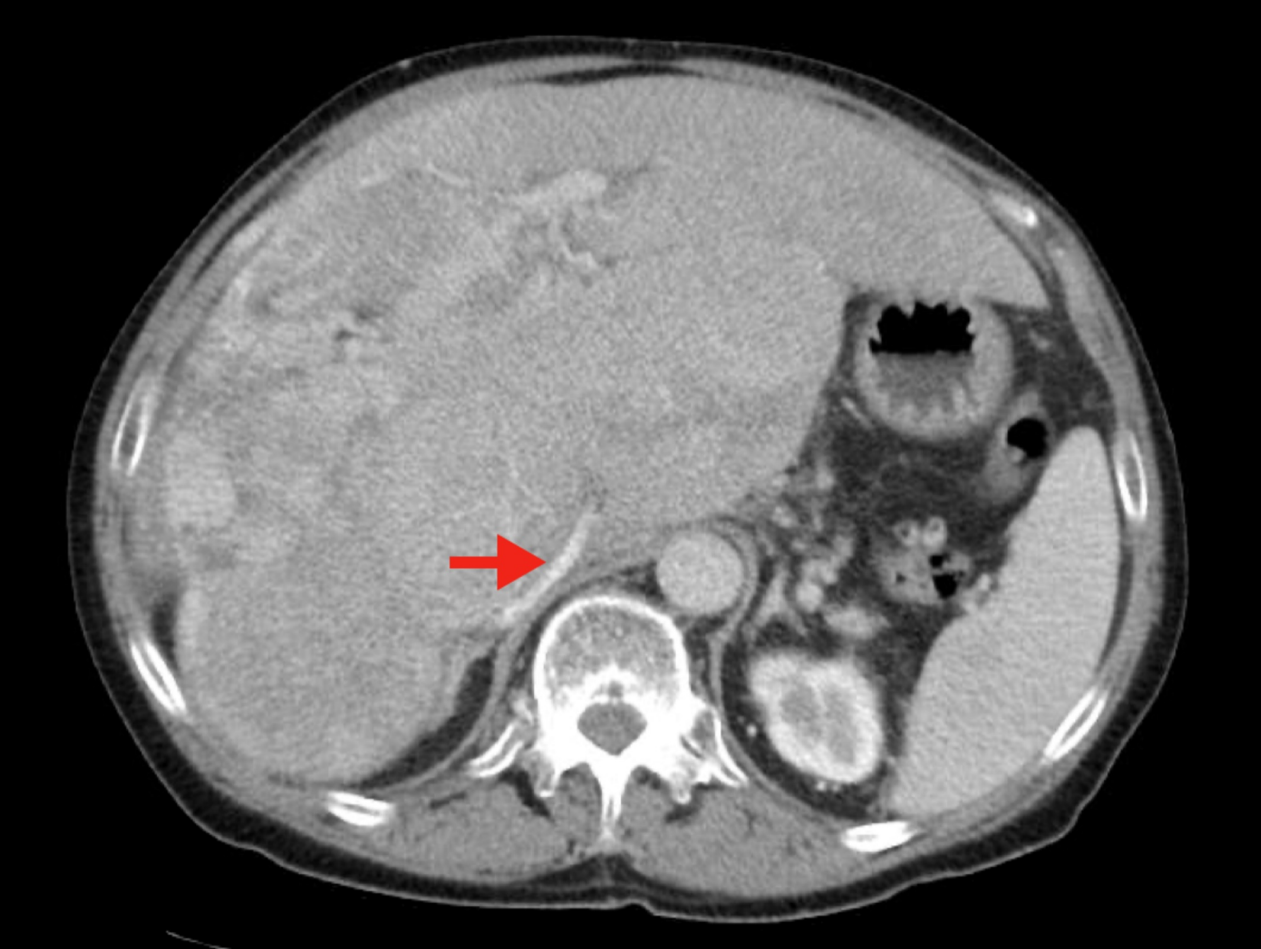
CT imaging 31 months after diagnosis demonstrating progression The liver is enlarged, with a persistent heterogeneous mass in the right hepatic lobe that is increased in size from previous studies. The mass causes mass effect and 'slitlike' appearance of the intrahepatic inferior vena cava (arrow).
